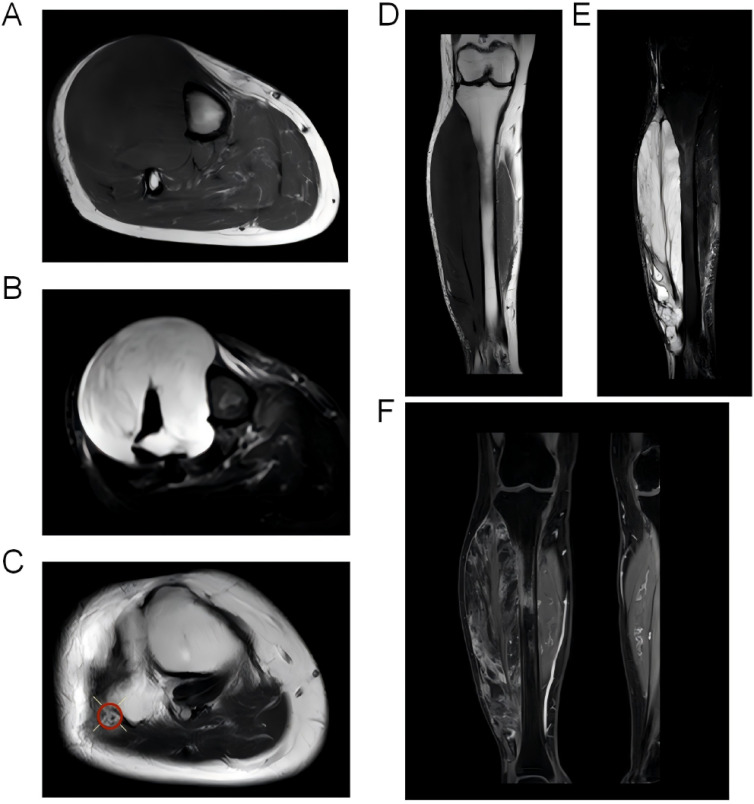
Preoperative MRI of the left calf showing an invasive lesion in the midstream tibia. The axial (A) T1-weighted image; (B) the “tail sign” can be clearly seen through the FS-weighted image; (C) T2-weighted image showing the common peroneal nerve to be invaded by the tumor at the location of the small head of the fibula. The coronal (D) T1-weighted, and (E) T2-weighted images. The sagittal (F) contrast-enhanced Dixon fat-water separation imaging.
